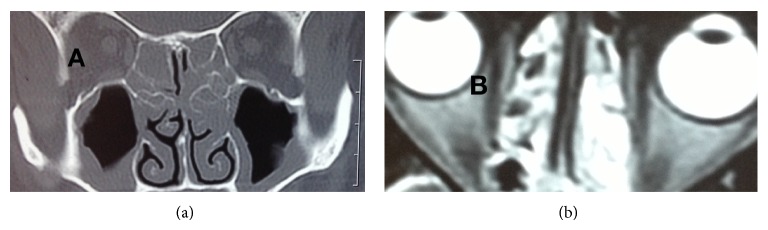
CT scan (a) and MRI scan (b) showing exuberant mucosal thickening of maxillary sinus and nose.
